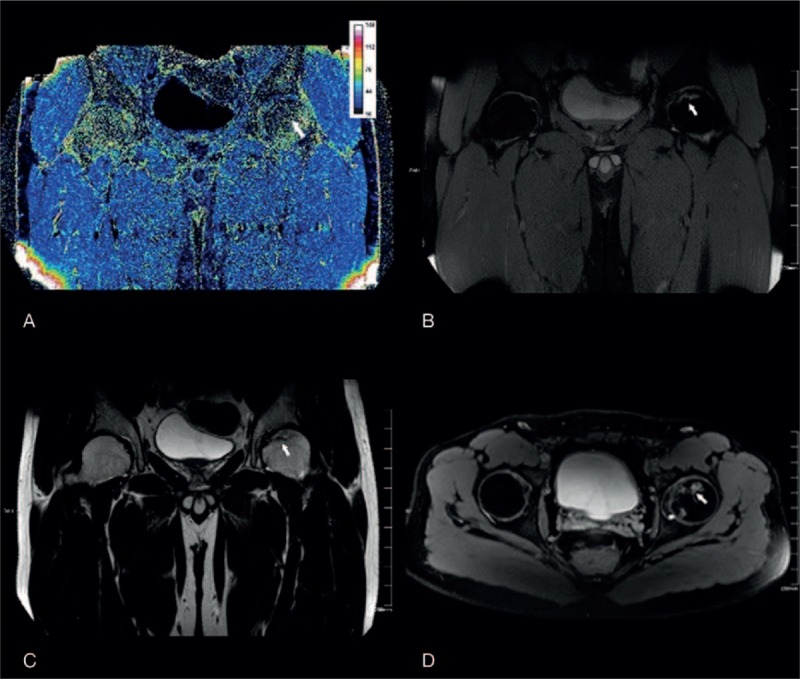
BOLD-MRI mapping and conventional MR image of a unilateral FHON patient. (A) R2∗ mapping reflected a lesion (white arrow) with the decreased value in the left side. The values of zones 1 to 3 declined. Conventional MRI (B–D, coronal PDW/SPAIR, coronal T2WI/TSE, axial T2WI/SPAIR) showed the lesion with a “double-line” sign on the T2WI sequence and a “serpentine-like” geographic border on the SPAIR sequence. The region of the lesion (white arrow) was obviously smaller than indicated by R2∗ mapping. BOLD-MRI = blood oxygen level-dependent MRI, FHON = femoral head osteonecrosis, R2∗ = total transverse relaxation time.
